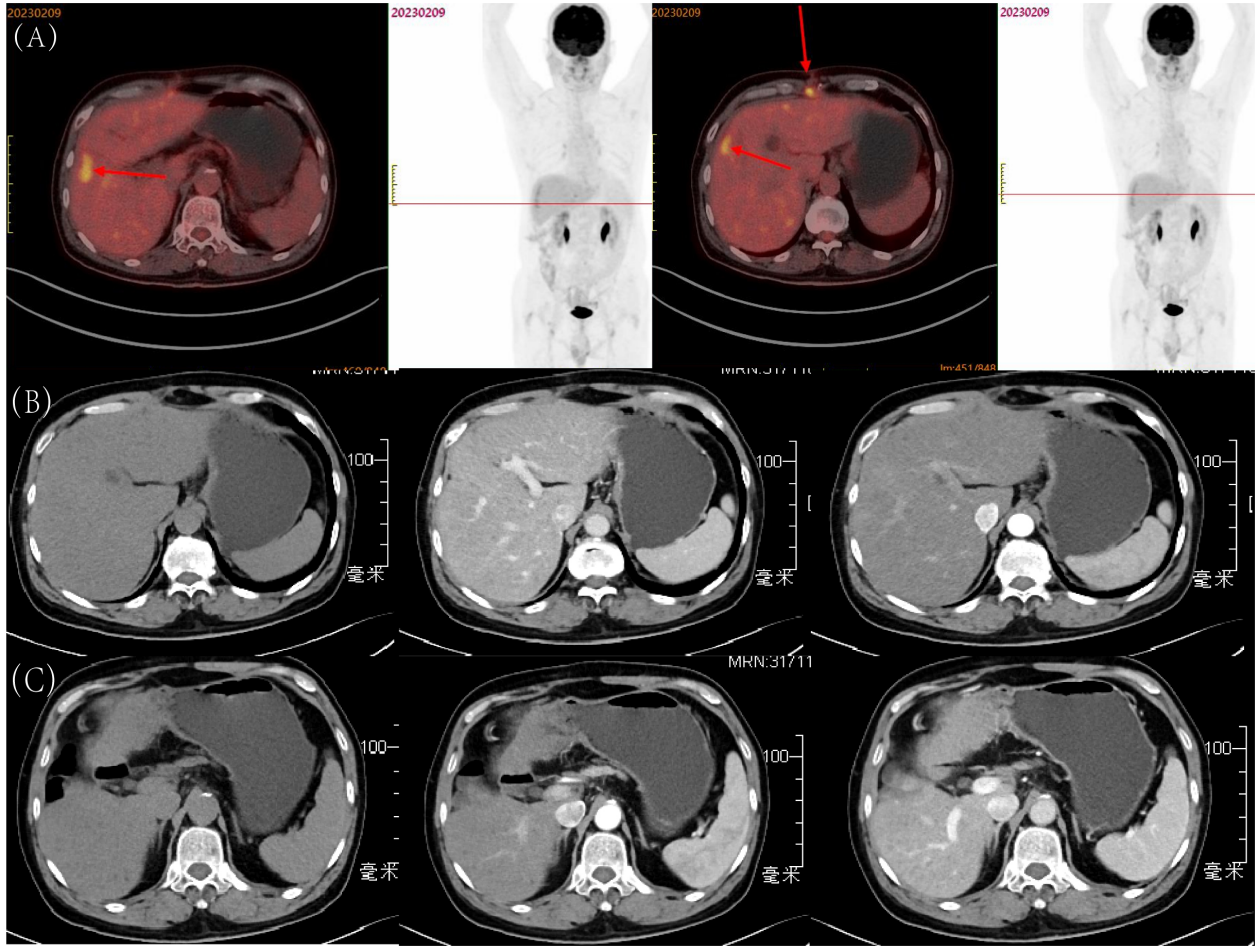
Postoperative Follow-up of the Patient: (A) Whole-body PET-CT examination three months after surgery; (B) Tumor in S8, eight months after surgery: before contrast, arterial phase, and portal venous phase; (C) Tumor in S5, eight months after surgery: before contrast, arterial phase, and portal venous phase.
